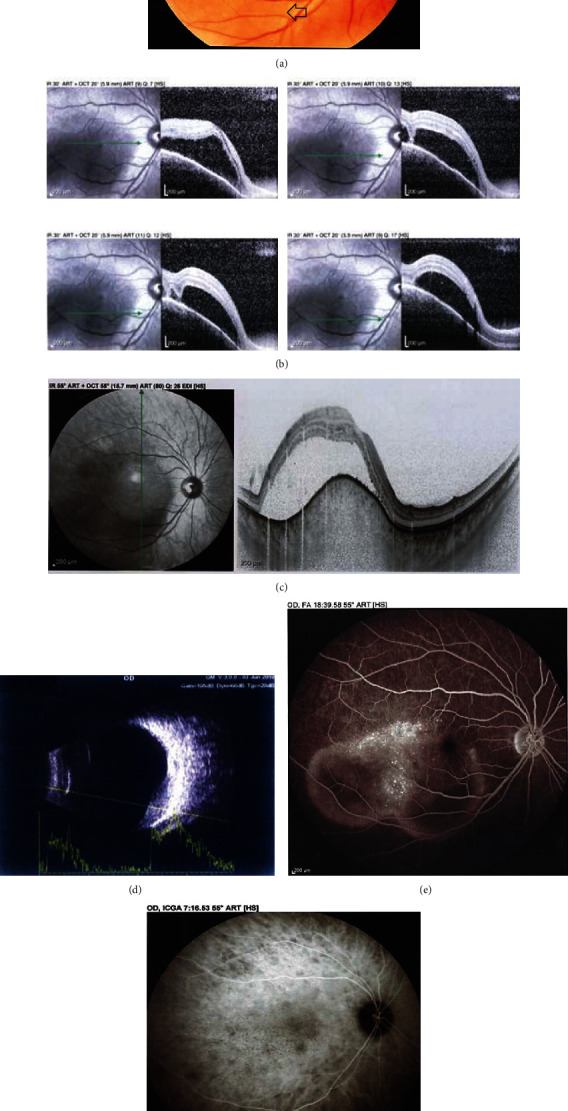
Initial examination of the right eye. Color fundus photo shows subretinal fluid (arrows) and elevated subretinal mass extending beyond the inferior arcade (a). Horizontal scans of optical coherence tomography (OCT) from the fovea to inferior vascular arcade shows subretinal fluid with a dome-shaped elevated choroidal mass in the macular area (b). Vertical scan of enhanced depth imaging optical coherence tomography shows a homogenous hyporeflective elevated lesion in the choroid (c). Ultrasonography shows a hyperechoic choroidal mass with medium to low internal reflectivity (d). Fluorescein angiography in the late phase shows the pooling of dye in the subretinal area and multiple hyperfluorescent dots over the lesion (e). Midphase indocyanine green angiography reveals multiple small, round, hypocyanescent dots in the posterior and midperipheral fundus (f).
